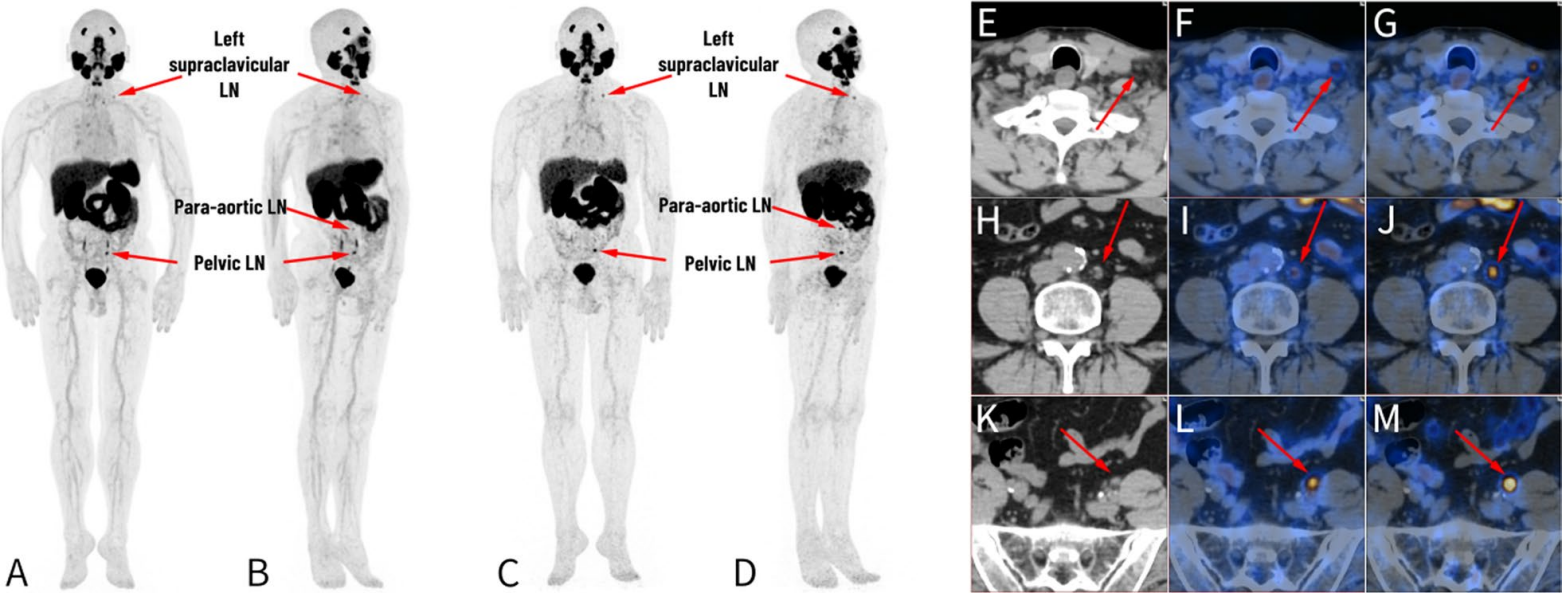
Delayed images on LFOV PET/CT showed the additional LNM in a 62-year-old primary PCa patient. Left supraclavicular LNM, para-aortic LNM and pelvic LNM could be detected on MIP of delayed LFOV images (tiles C–D), whereas MIP of early images could not make the clinically sufficient diagnoses (tiles A–B). Left supraclavicular small LNM (tiles C–D, G) was more distinguishable in the delayed image due to the increased SUV_max_ value (3.3 at 1 h p.i. vs. 5.3 at 3 h p.i.), which was confirmed as metastasis by LN biopsy. The para-aortic small LNM was faintly visible in the early image (tiles B, I), while it showed the increased SUV_max_ value (3.3 at 1 h p.i. vs. 11.1 at 3 h p.i.) in the delayed image (tiles D, J). Multiple foci with avid [^68^ Ga]Ga-PSMA activity were demonstrated along the bilateral urinary tracts in early images (tiles A, B, L), whereas only one intense [^68^ Ga]Ga-PSMA activity was observed in the left pelvic region in delayed images, with a SUVmax value of 15.2 (tiles C, D, M), the CT image showed a small nodular lesion with a size of 7.5*5.5 mm, which was verified as LNM. Without the urinary urine activity interference, the pelvic LNM was more visible on delayed image
